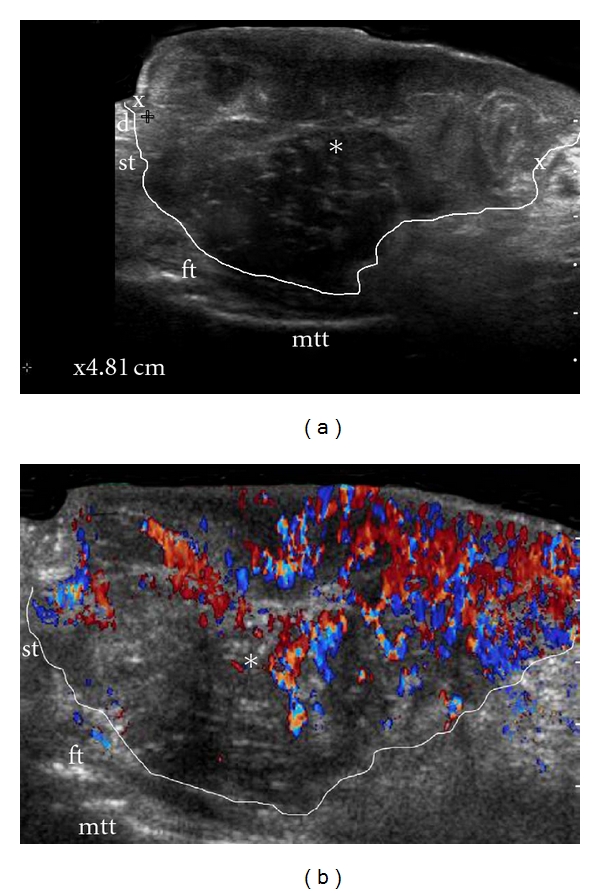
Bulky melanoma at the plantar region. (a) Ultrasound (longitudinal view) shows heterogeneous mass (∗, and outlined) infiltrating dermis and subcutaneous tissue, almost reaching the flexor tendon (fl) close to the second metatarsal bone (mtt). (b) Color Doppler ultrasound shows highly increased blood flow covering most parts of the lesion.
